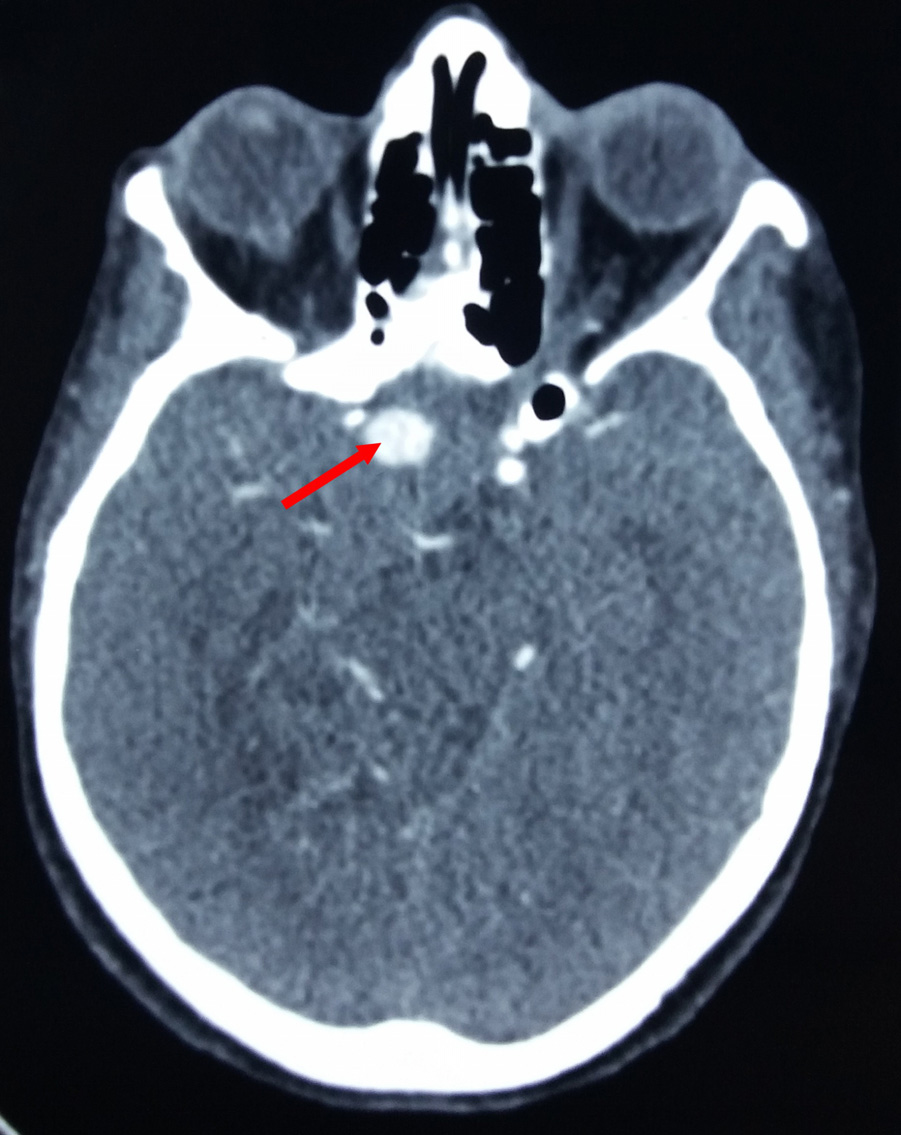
CT angiography showing the saccular aneurysm (red arrow) that measured 1.7 cm in maximal dimension. Enhancement was similar to the arterial network and there was a significant communication with the internal cavernous carotid artery. No additional aneurysms were identified and there was no arterial occlusion or hemodynamically significant narrowing (Color version of the figure is available online.)
